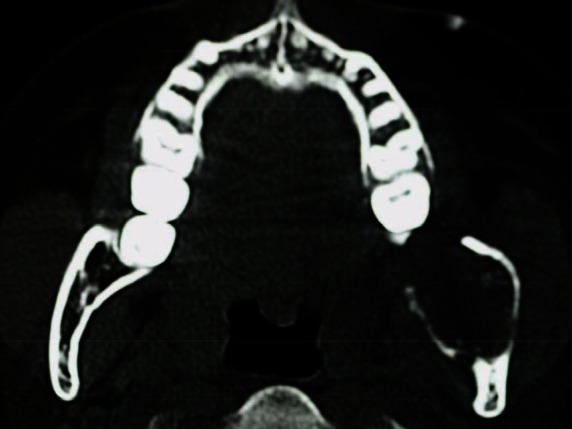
CT scan showing expansion and perforation of buccal and lingual cortical plates
